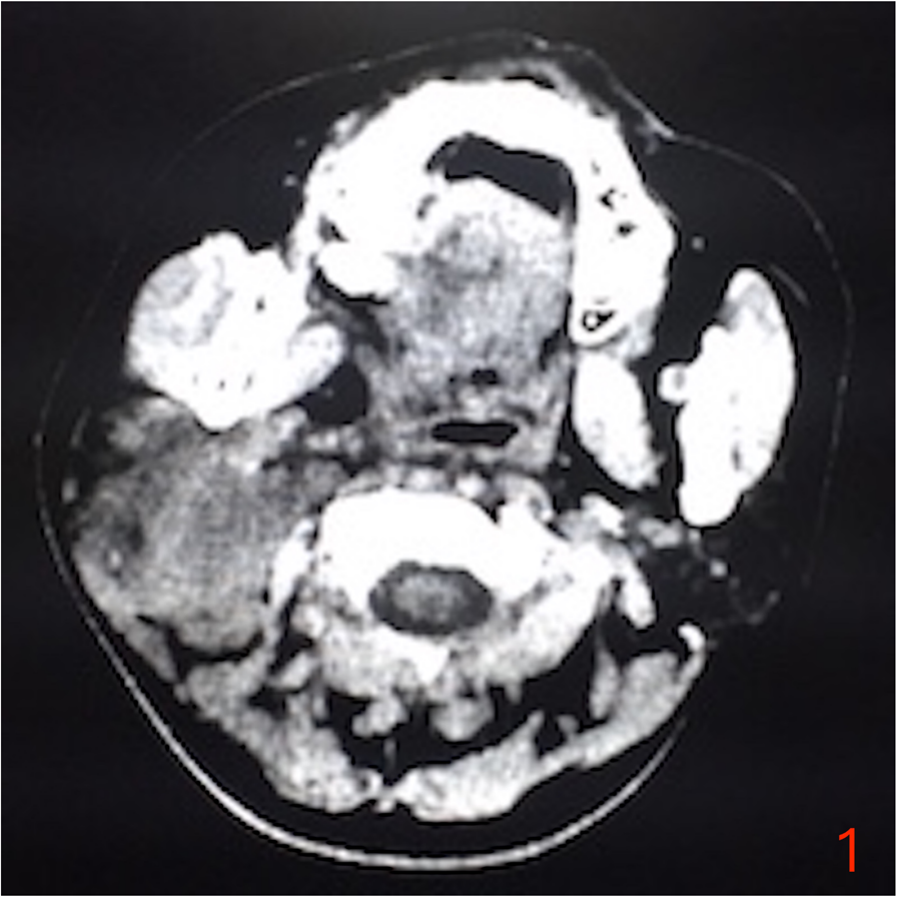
Enhanced neck CT showing multiple swollen lymph nodes of varying sizes fused into a 54 × 39 × 83 mm mass with irregular edges and heterogeneous enhancement
